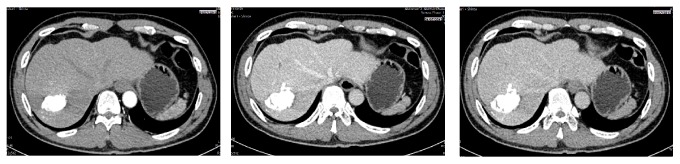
Arterial, venous, and delayed phase of Abdominal CT Scan after second DEB-TACE (May 2013).
